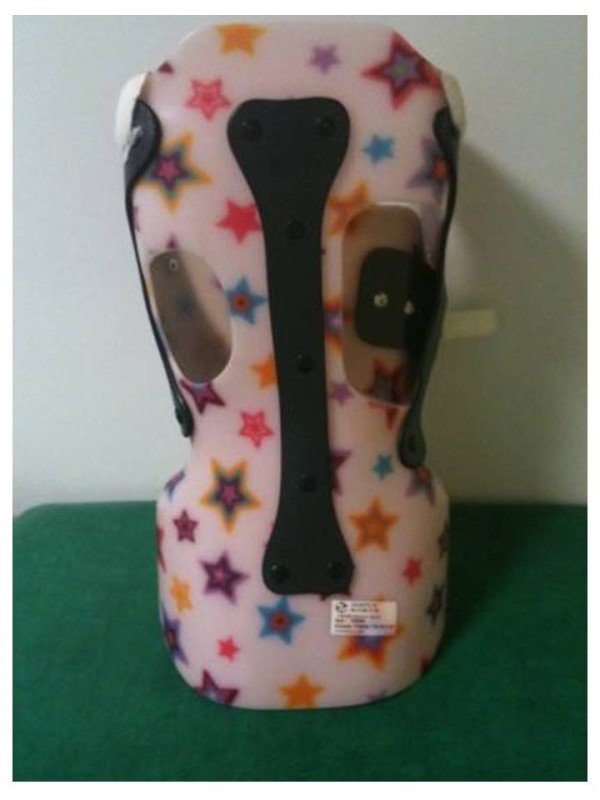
Face and profile x-rays without brace. Thoraco-lumbar scoliosis.
